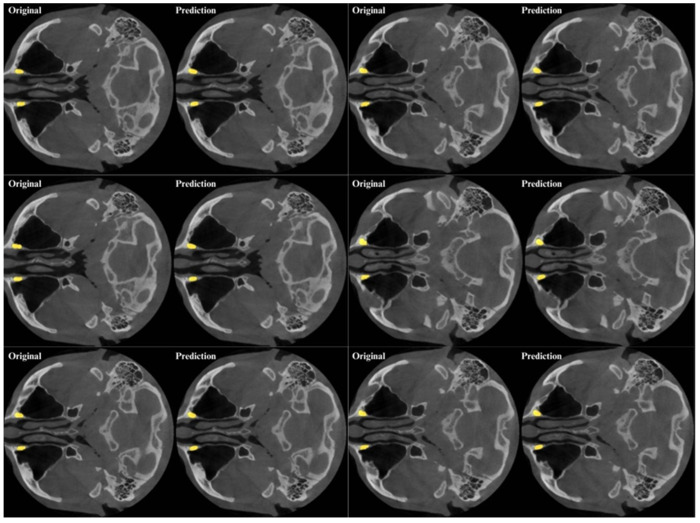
Automatic segmentation of nasolacrimal canal using artificial intelligence model in axial CBCT slices.
